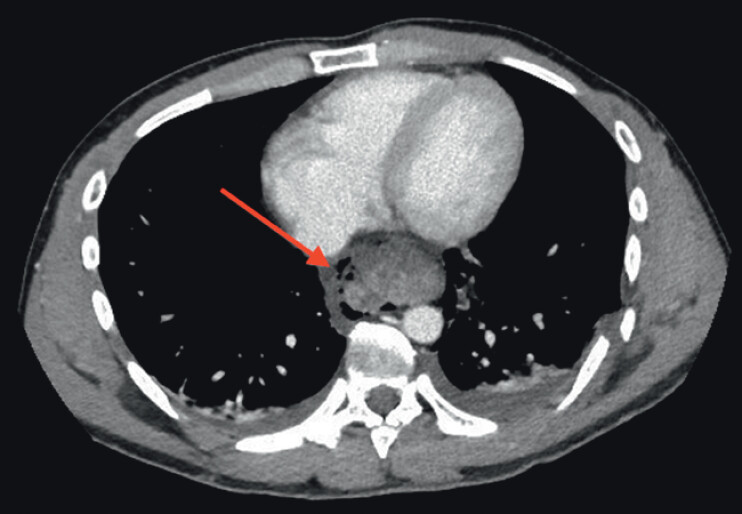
Esophageal perforation on computed tomography scan.
